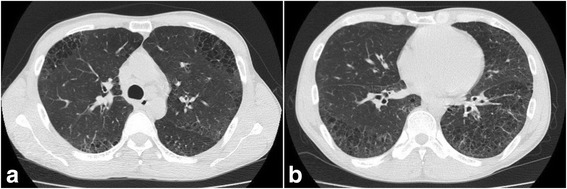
Chest computed tomography image of Case 2. a In both the upper lungs, cystic lesions with bronchiectasis were observed. b Peripheral reticulations and cystic lesions were found in both lower lobes
